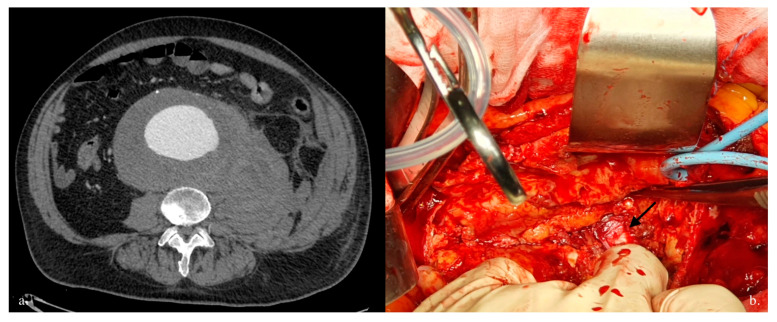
(a) CTA shows a postero-lateral AAA rupture with retroperitoneal hematoma. (b) The picture shows an open surgical repair of an RAAA with vertebral exposure (black arrow). CTA, Computed Tomography Angiography; AAA, abdominal aortic aneurysm; RAAA, ruptured abdominal aortic aneurysm.
